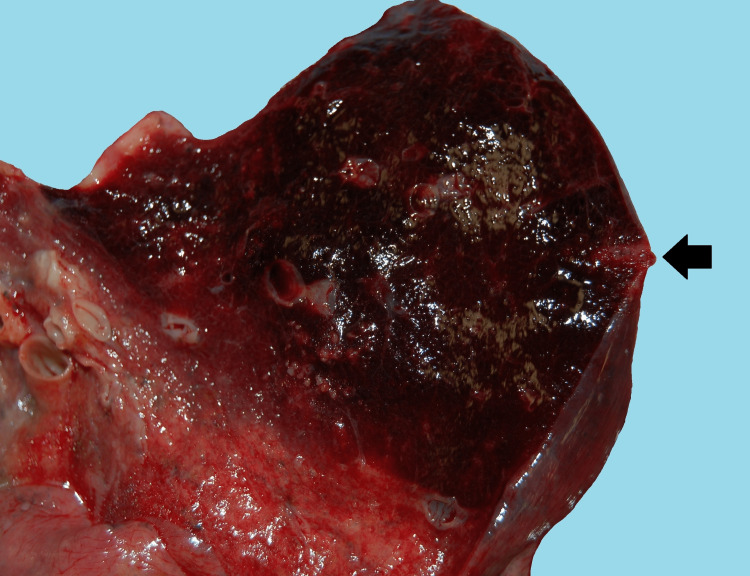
A case example of a lung biopsy as a "probable" contributor to death Laceration and intraparenchymal hemorrhage at the site of a right lung transthoracic biopsy (arrow) were performed approximately four days prior to death. This finding was associated with a 3L hemothorax. The 74-year-old patient was admitted for anticoagulation therapy for pulmonary emboli, and a biopsy was performed for a suspicious lung nodule seen in imaging studies. The biopsy did not reveal evidence of malignancy, and post-mortem examination of the lung did not identify a mass lesion.
